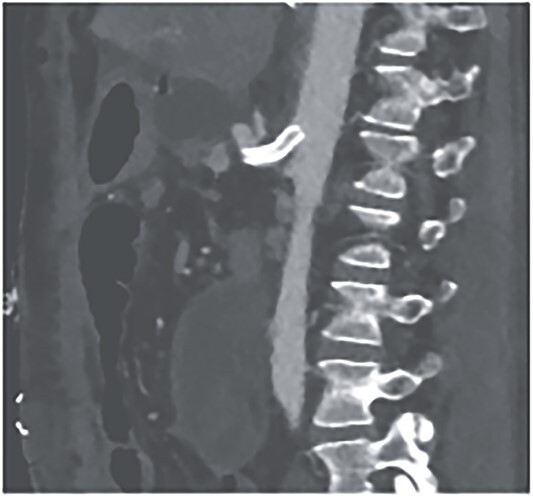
CTA showing successful intravascular sentient of celiac trunk.
